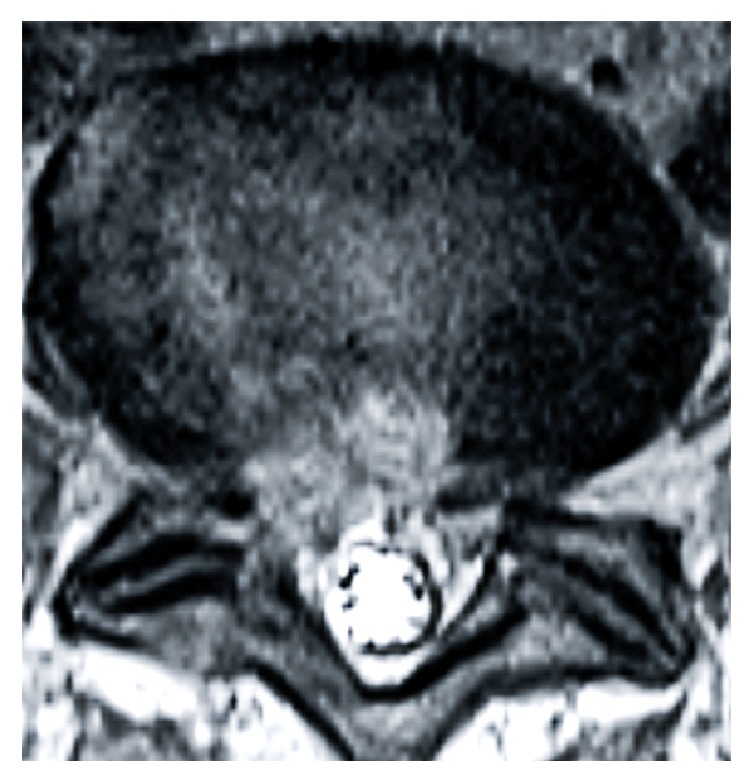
Postoperative MRI results indicating complete removal of the herniated disc material.
